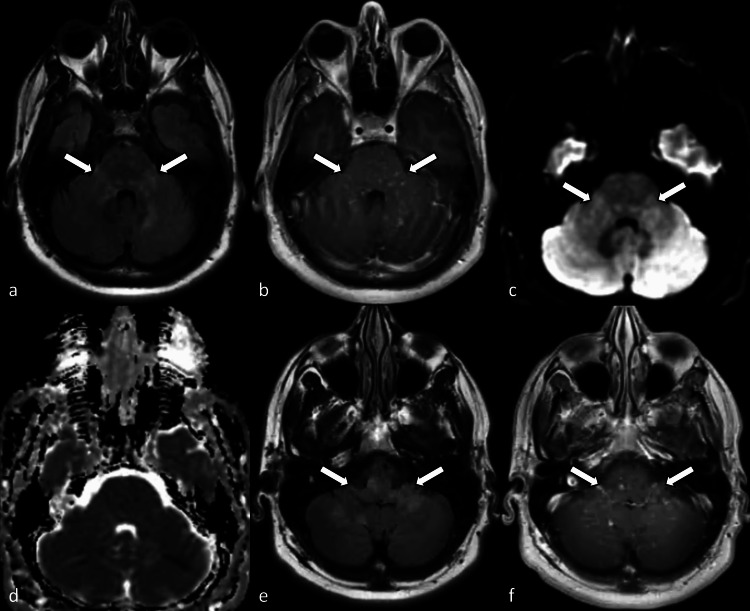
Brain MRI a, e: Fluid-attenuated inversion recovery (FLAIR) axial images, b, f: contrast-enhanced T1-weighted axial images, c: diffusion-weighted axial image, d: apparent diffusion coefficient map. Brain MRI shows multiple nodular FLAIR hyperintensity in the pons, bilateral middle cerebellar peduncles, cerebellar hemispheres, and medullar oblongata with corresponding contrast enhancement and diffusion-weighted hyperintensity without definite diffusion restriction (a-f, arrows).
